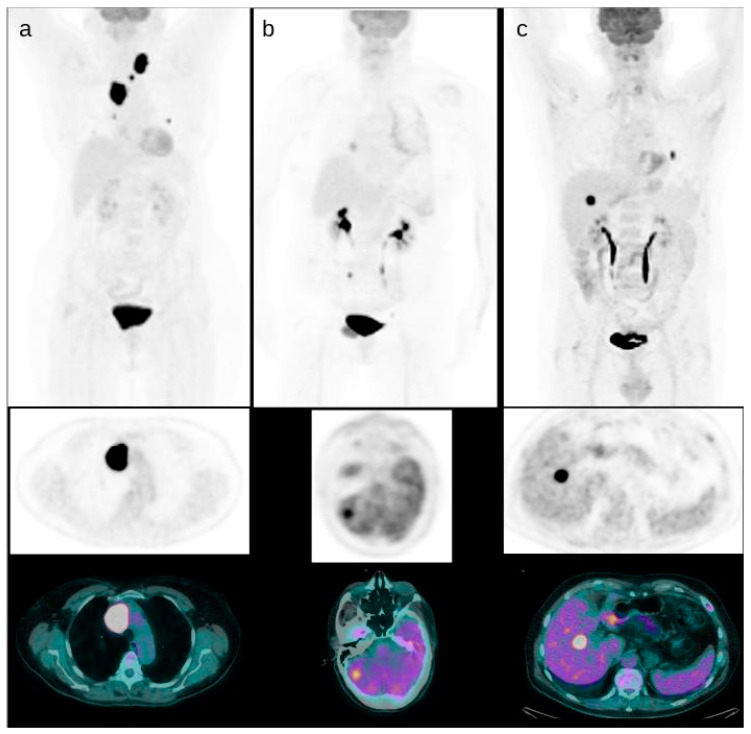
Some examples of DTC patients with positive PET/CT. A patient with mediastinal and thoracic FDG positive disease and the lesion with higher uptake in the upper mediastinum ((a), SUVmax 55). A patient with brain metastases detected by PET/CT with uptake higher than the surrounding brain parenchyma ((b), SUVmax 20). A patient with liver metastases ((c), SUVmax 35).
